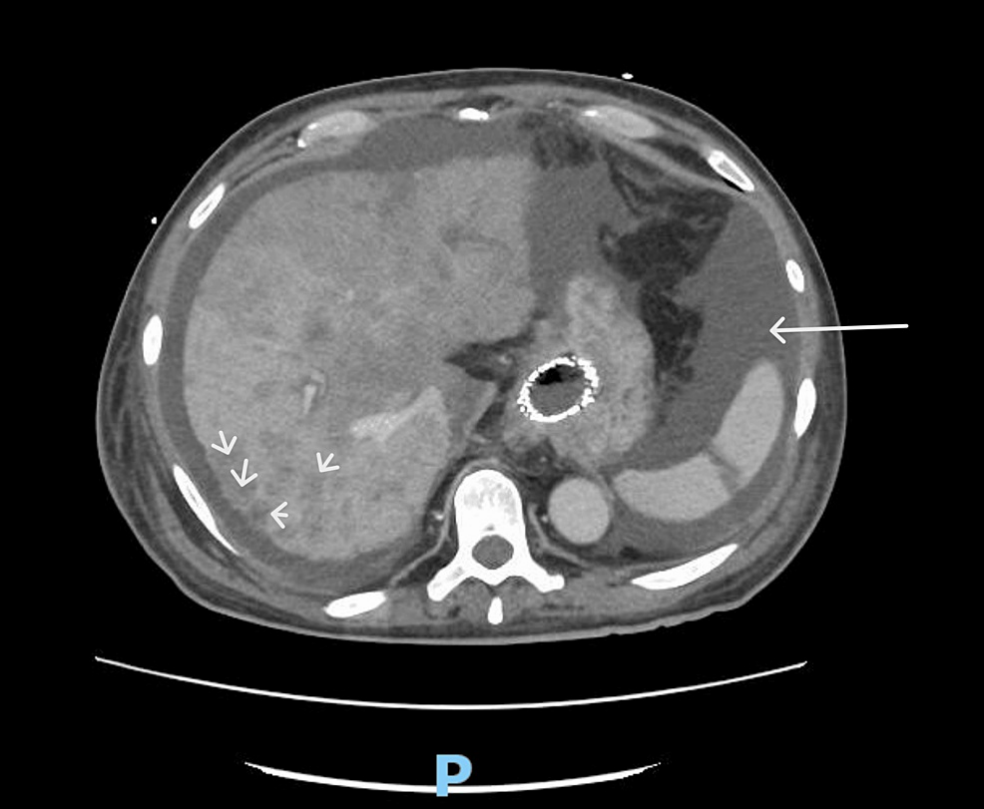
CT Abdomen with IV contrast involving enlarged spleen and multiple hepatic lesions
